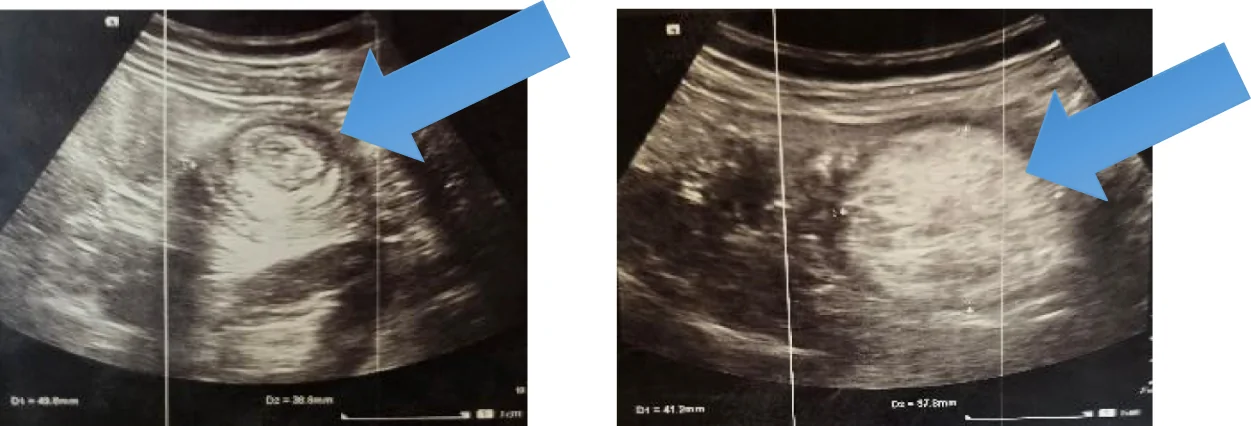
Abdominal ultrasound: Target-like lesion measuring 49 × 38.8 mm in left upper quadrant; pseudokidney sign on longitudinal view; bright lesion 4.1 × 3.7 cm inside bowel just beyond intussusception.
